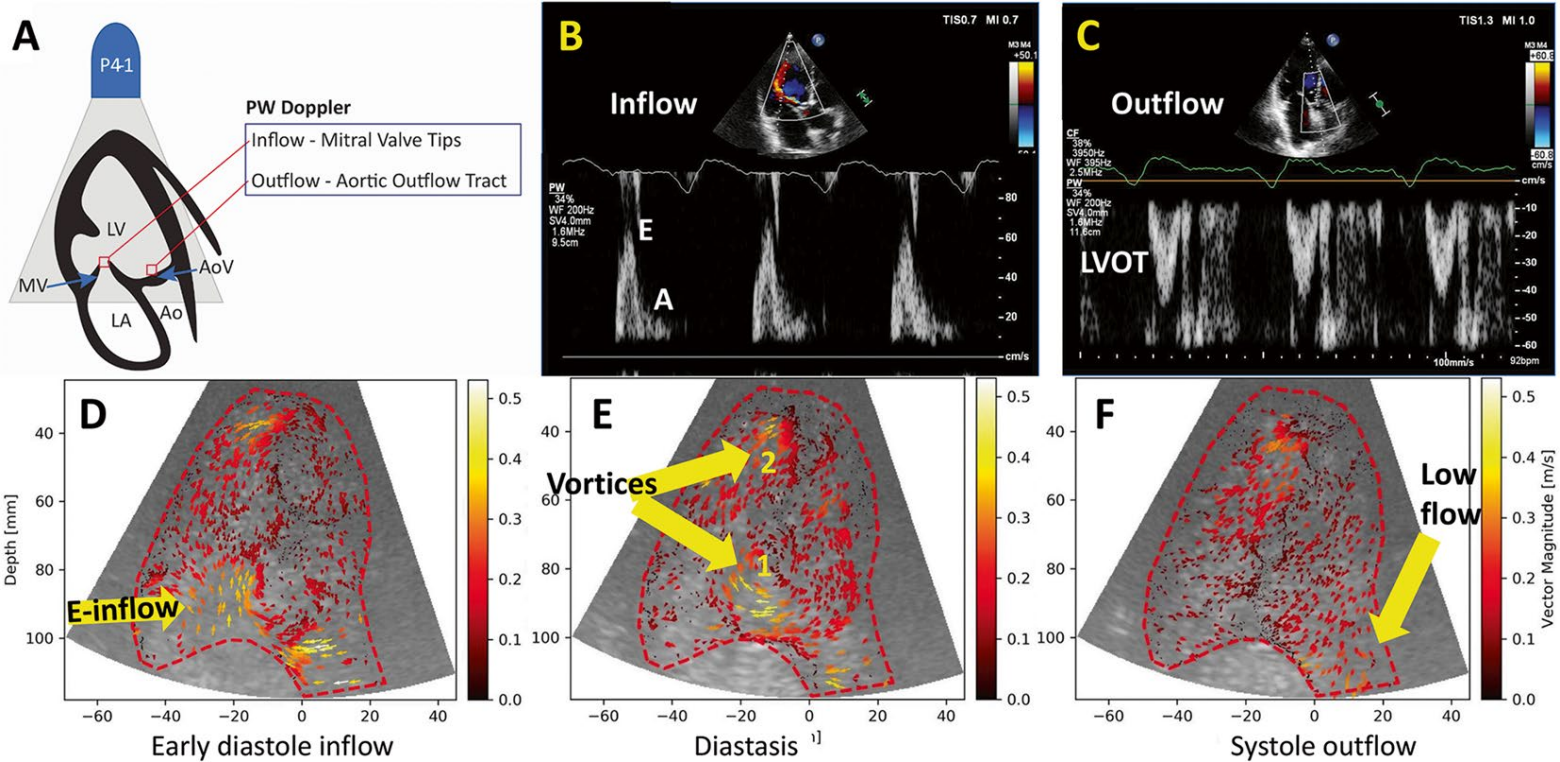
Image acquisition. A: apical long axis view with the position of the Doppler and echoPIV interrogation points; B: PW Doppler of the inflow in a heart failure patient. Early diastolic flow (E wave) was measured in this study, the post-atrial contraction flow (A wave) was not considered; C: left ventricle outflow tract (LVOT) PW Doppler flow in the same heart failure patient, demonstrating low output (low velocity); D: echoPIV tracking of the early diastolic inflow in the same patient; E: mid-diastolic vortex formation in the LV. Two main diastolic vortices (1,2) can be seen; F: low velocity outflow, confirmed by the PW Doppler (C)
